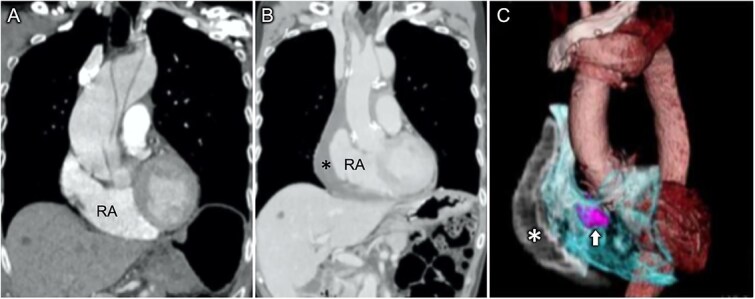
Contrast-enhanced CT showing the anatomical relationship between the ascending aorta and the right atrium. Postoperative dilatation and elongation of the ascending aorta, along with pericardial effusion, may contribute to right atrial compression and facilitate right-to-left shunting. Arrow, impending paradoxical embolism; ^*^, pericardial effusion; RA, right atrium.
